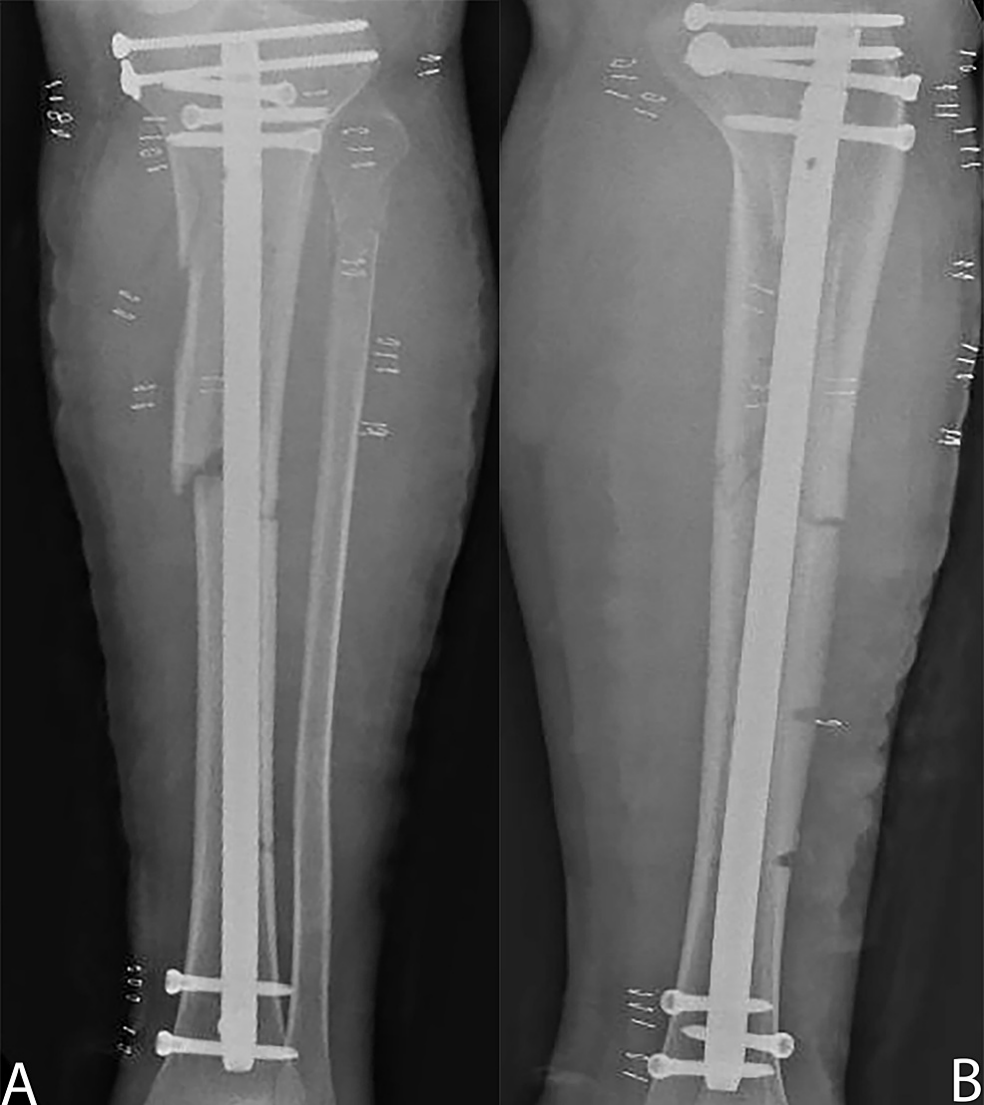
Definitive treatment. A: Post-operative anteroposterior radiograph demonstrating definitive fixation of tibial fracture by an intramedullary nail. B: Post-operative lateral radiograph demonstrating definitive fixation of tibial fracture by an intramedullary nail.
